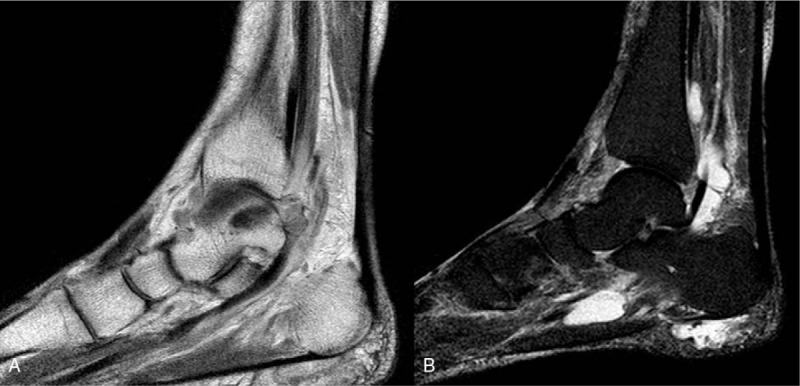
Sagittal T1-magnetic resonance imaging (MRI) image of tophus mass on the tarsal tunnel area. A, Sagittal T2 MRI image of tendonitis with fluid collection around flexor hallucis longus (FHL) tendon, adductor hallucis longus, and insertion of the plantar fascia.
